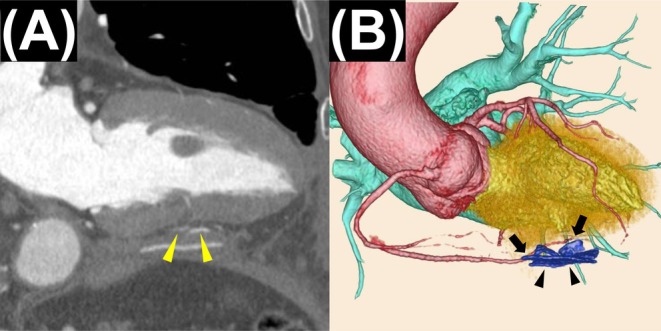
Postoperative CT images. (A) Long‐axis view demonstrating complete exclusion of the aneurysm cavity (arrowheads). (B) Three‐dimensional reconstruction showing complete exclusion of the aneurysm cavity (arrowheads) and preserved course of the right coronary artery to its distal portion (arrows).
